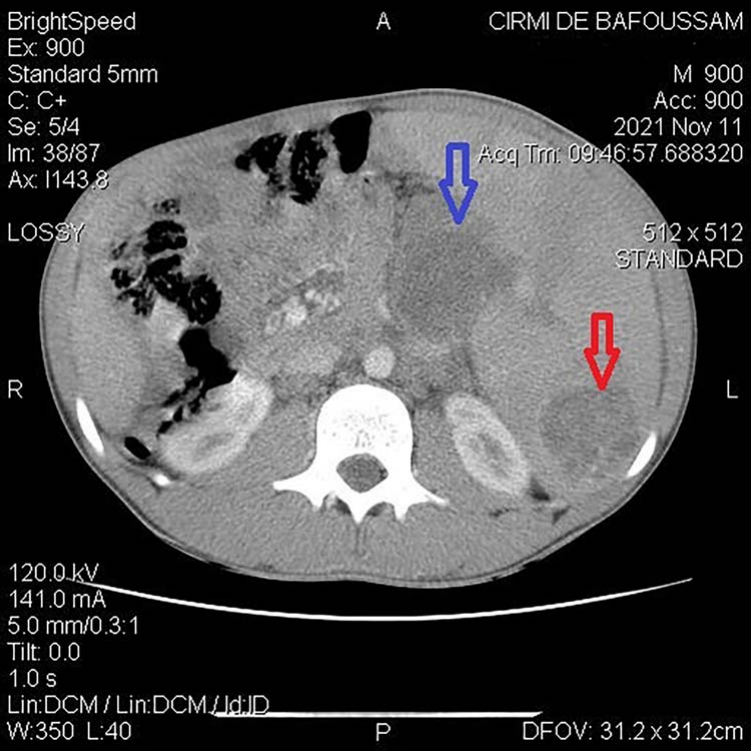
Abdominal CT demonstrating a cystic mass in tail of the pancreas (top arrow) and the tip of the spleen (bottom arrow).
